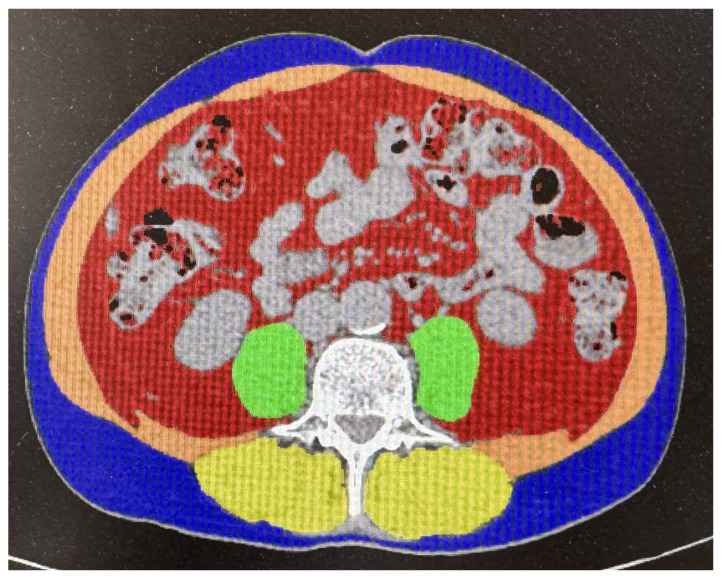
CT evaluation of the cross-sectional area of lumbar skeletal muscles at the L3 level using Synapse 3D. Muscles are highlighted in yellow and orange.
